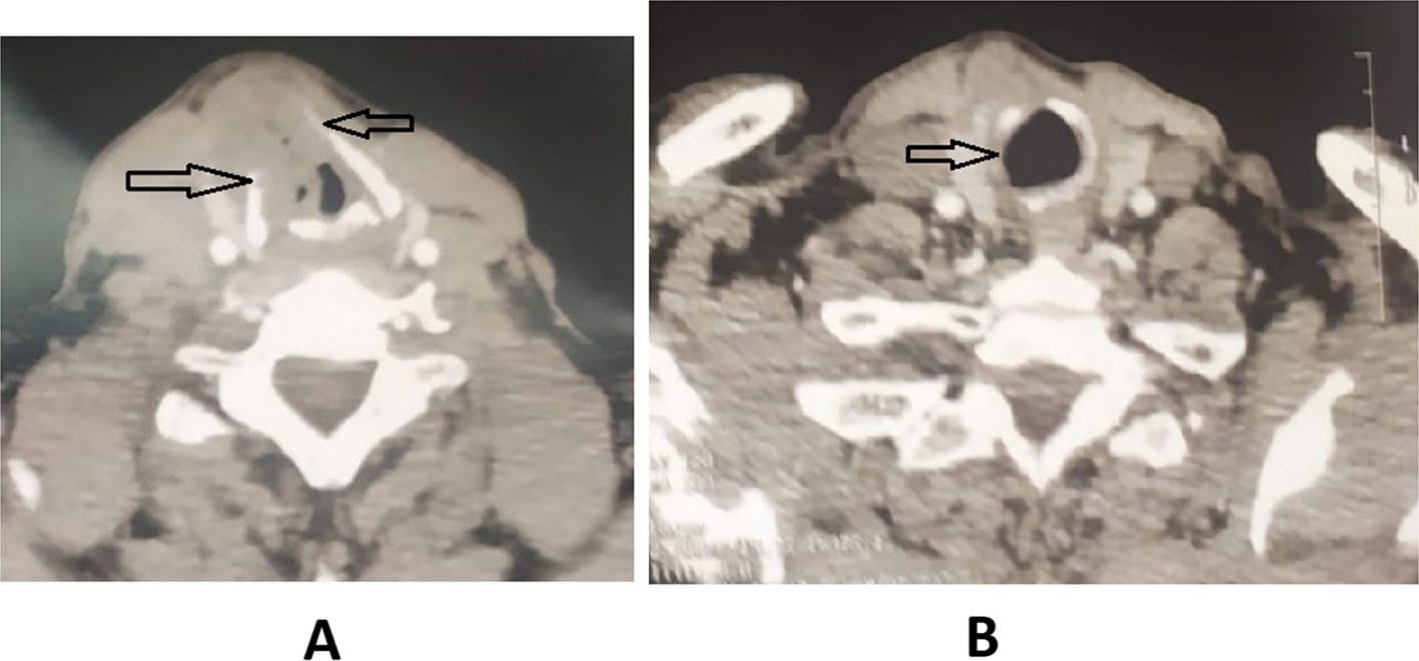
CT scan showing laryngeal cartilages invasion. A Thyroid cartilage invasion (arrows). B Cricoid cartilage invasion (arrow)
